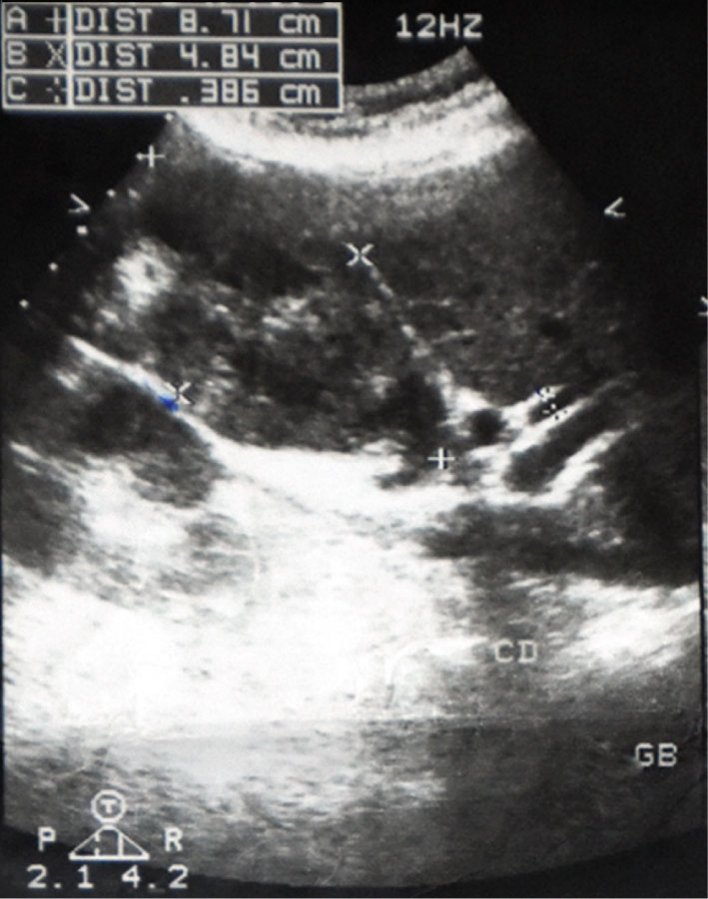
Transabdominal ultrasonography.
